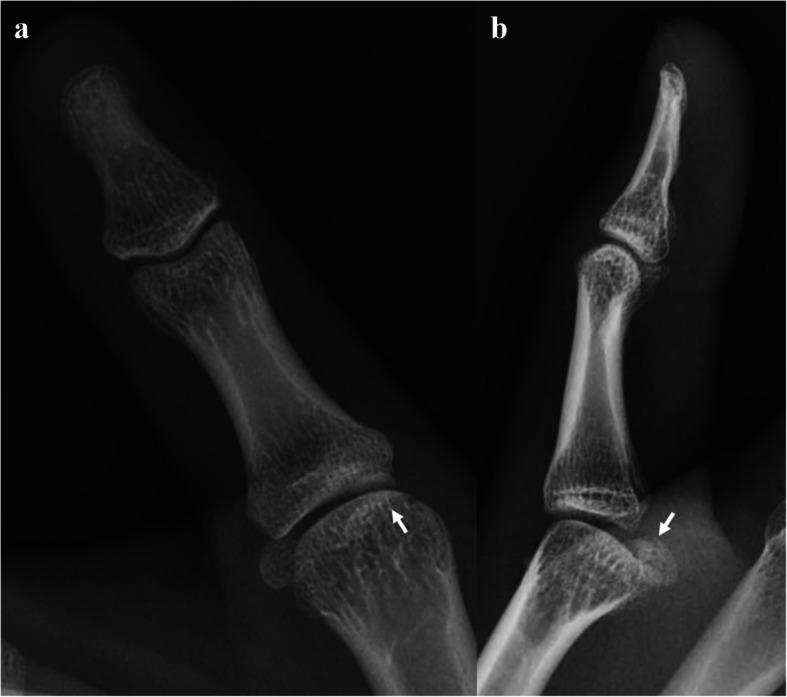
Anteroposterior (a) and lateral (b) radiographs of the right thumb. The lateral radiograph shows a hyperextension posture of the metacarpal joint at approximately 30°. The abnormal location of the radial sesamoid (arrow) is difficult to recognize on radiographs because of bony overlaps
